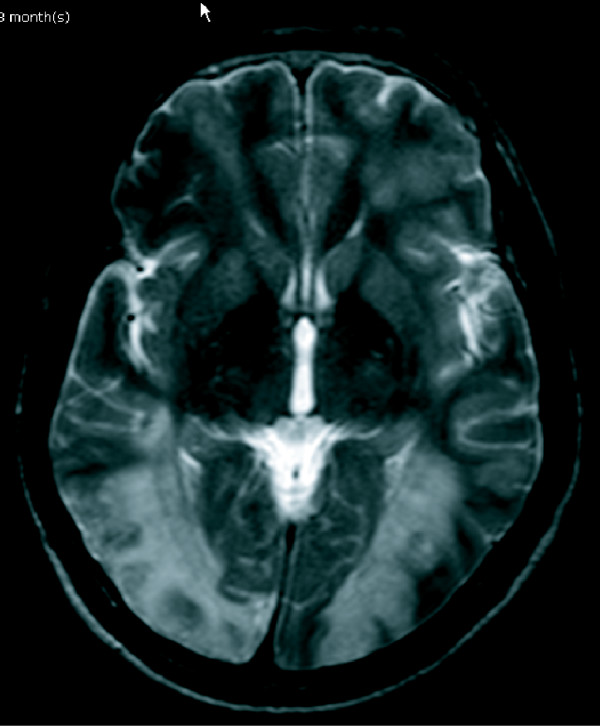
MRI scan of the brain demonstrating diffuse multi-stage intraparenchymal infarction and hemorrhage at both cerebral and cerebellar hemispheres, initially considered to be vasculitis.
